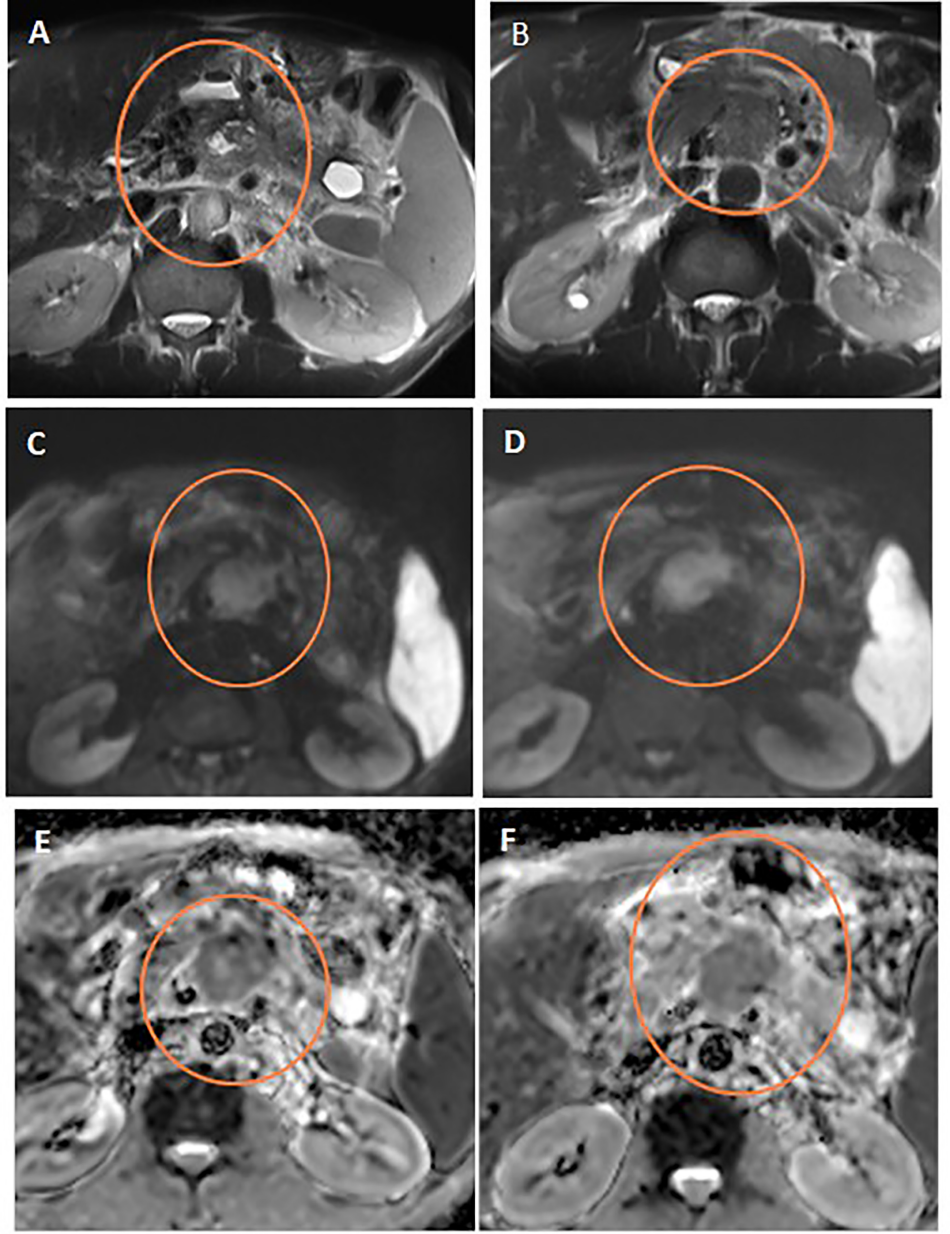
Discordant response between ADC and RECIST. Axial T2WI (A) shows stationary size of the pancreatic mass after neoadjuvant therapy compared to initial axial T2WI (B). Note the central cystic change of the mass of necrosis. Post-treatment and initial DWIs (C, D, respectively) show corresponding restricted DWI of the mass. Post-treatment and initial ADC maps (E, F, respectively) show regression of the ADC values of the mass on post-treatment study compared to initial one.
